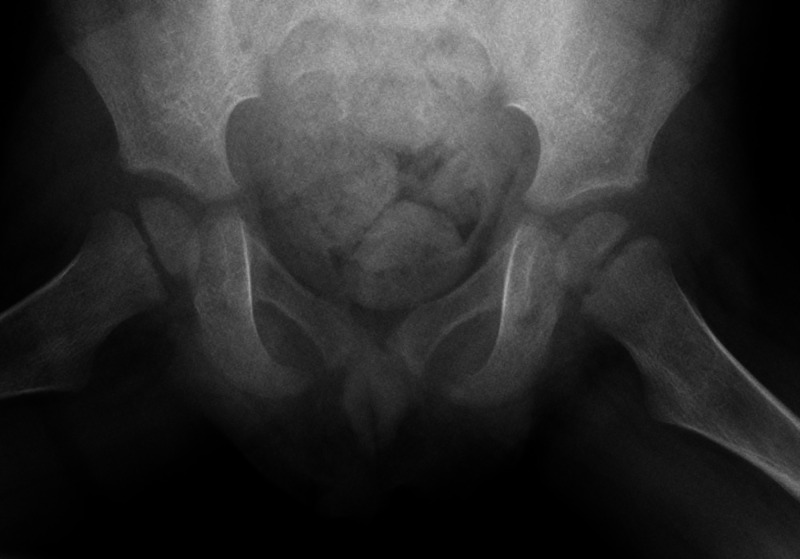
X-ray of the pelvis (frog leg lateral) from initial presentation to hospital
